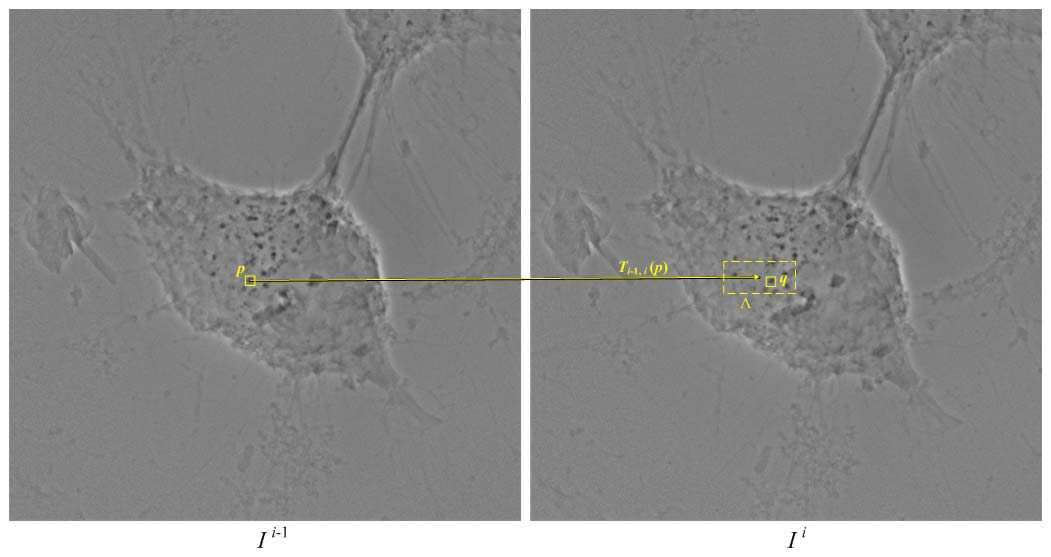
The feature tracking between two X-ray projection images *I^i^*
